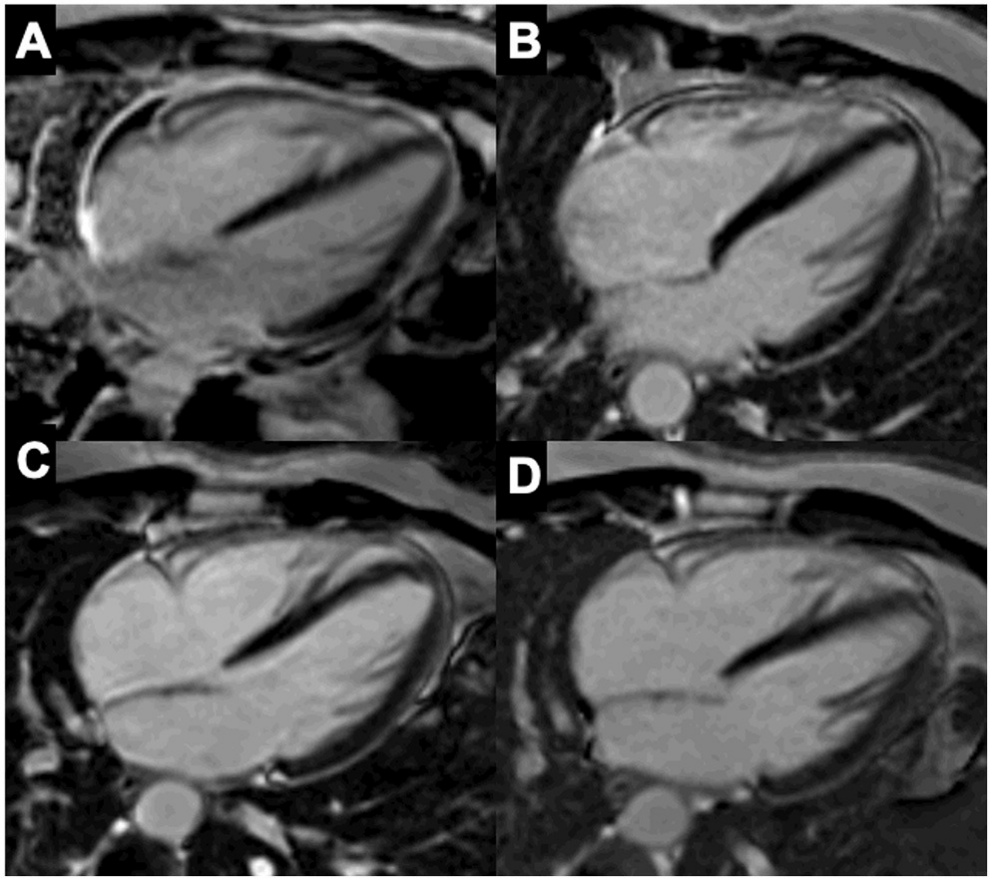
Representative patient with pericarditis and resolution of the pericardial inflammation on sequential CMRI scans. Four-chamber late gadolinium enhancement (LGE) PSIR cardiac MRI images of a 35-year-old male patient who presented with recurrent idiopathic pericarditis under NSAIDs and colchicine. (A) At presentation, there was diffuse pericardial thickening and LGE, and presence of pericardial effusion. High-dose corticosteroids (prednisolone 40 mg once daily) with taper schedule and azathioprine were initiated. (B) Re-evaluation after 3 months showed regression of pericardial LGE and disappearance of pericardial effusion. This allowed to further decrease the dose of steroids. (C) Follow-up cardiac MRI 5-months later showed near resolution of pericardial LGE. A low dose of steroids (prednisolone 4 mg once daily) was maintained. (D) Control after 1 year showed a normal pericardium without LGE. Image courtesy of Bernard Paelinck (Antwerp University Hospital).
